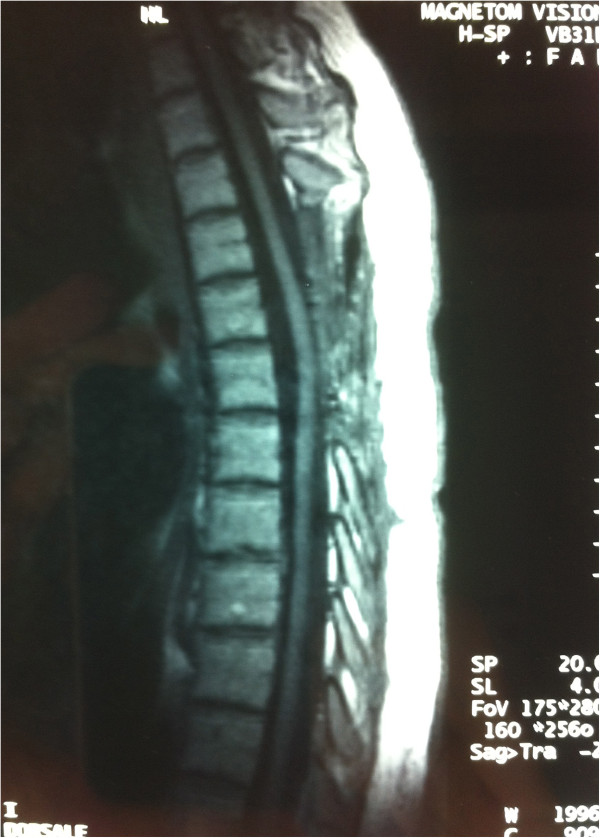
T1-weighted sagittal magnetic resonance image one month later, showing no evidence of residual lesion.
